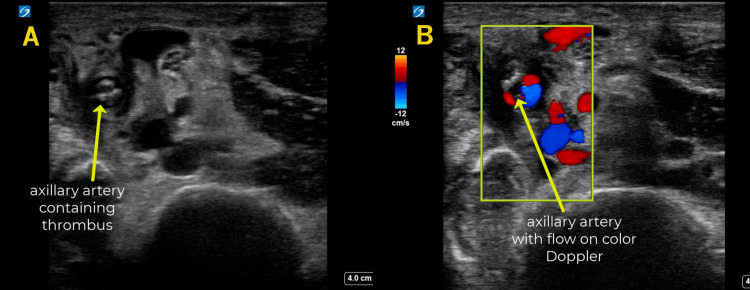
Partial Axillary Embolic Disease In these images taken from the proximal upper arm, the dilated axillary artery is seen containing irregular, echogenic thrombus (A); however, color Doppler evaluation confirms the occlusion is incomplete (B).
